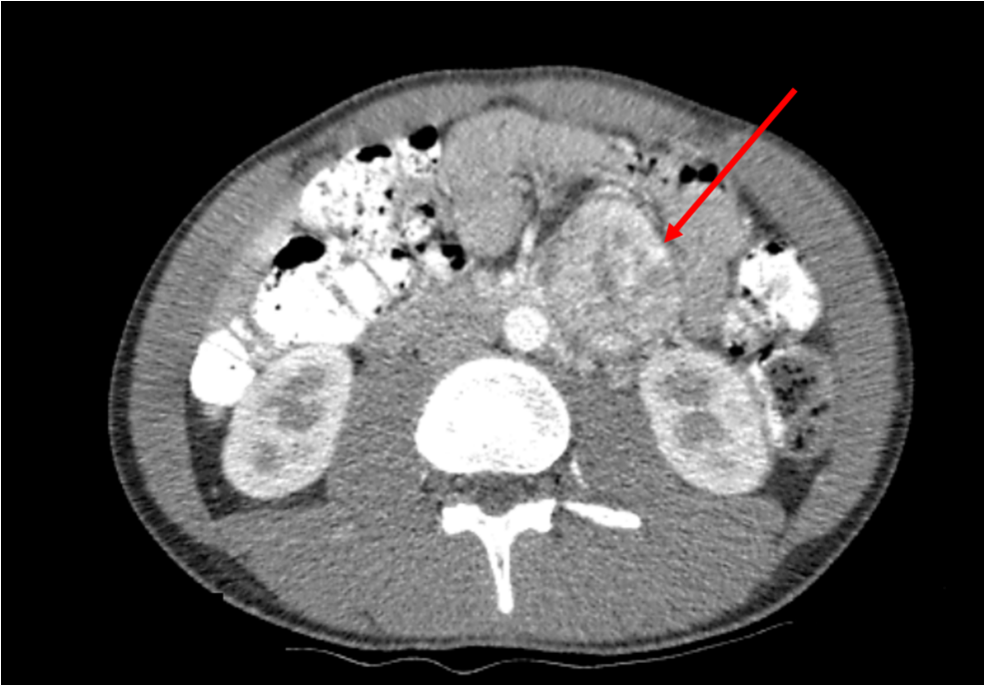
Abdominal CT scan demonstrating a hypervascular para-aortic mass of 7 cm with central necrosis, located caudal to the left kidney
